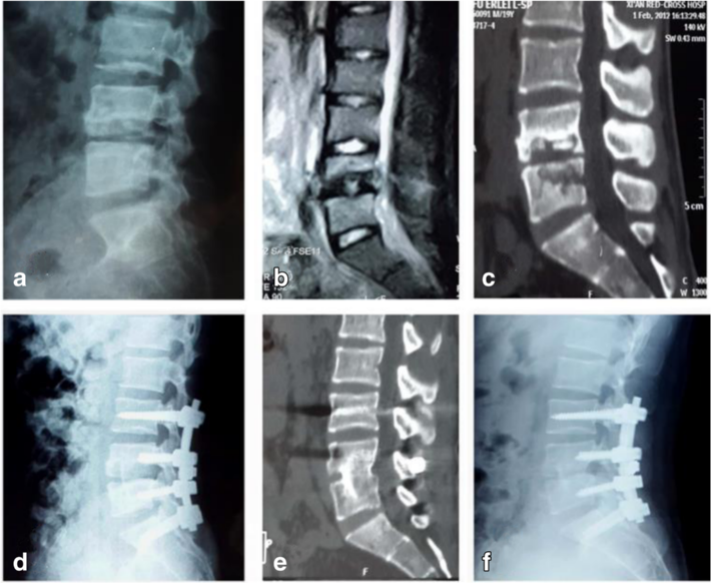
Images from a 21-year-old man with lumbar spinal tuberculosis who underwent one-stage posterior debridement, bone grafting, and instrumentation. a–c Preoperative X-ray, MR, and CT images showing the destruction of L4 and L5 and paravertebral abscess formation. d Postoperative lateral X-ray showing fixation of L3–S1. e, f At the 24-month follow-up examination, fixation and interbody vertebral fusion were satisfactory, with no sign of tuberculosis recurrence
